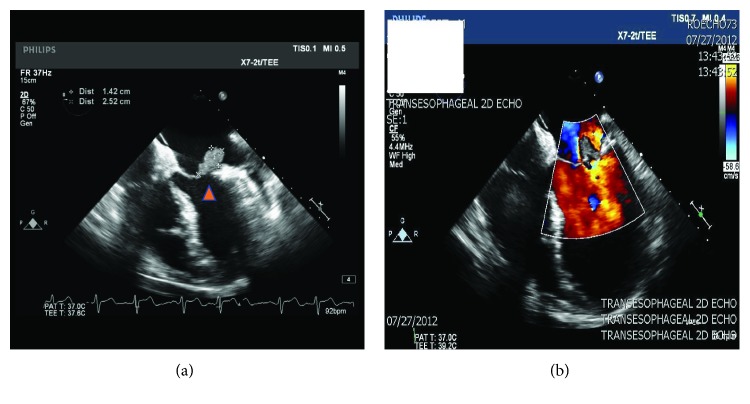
(a) TEE image of vegetation on posterior leaflet of mitral valve, measuring “1.42 cm × 2.52 cm” (arrowhead). (b) TEE image with evidence of blood flow regurgitation with Doppler into the left atrium in the setting of the mitral valve posterior leaflet vegetation.
